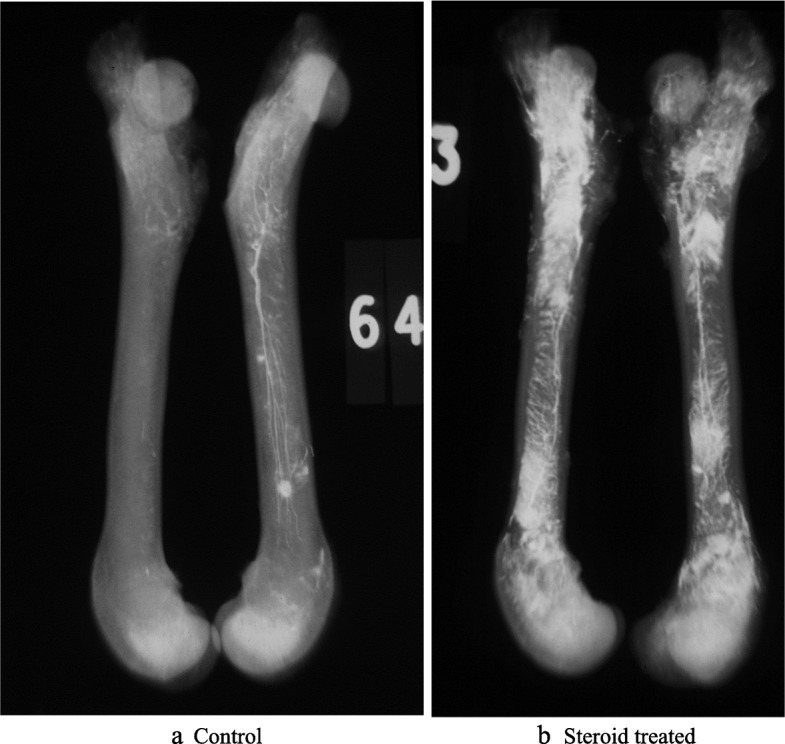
a and b showing examples of femoral angiogram microradiographs. The left radiograph 5a shows a pair of femora from a control barium angiogram. The right femoral pair 5b were from a steroid treated subject. After steroid treatment there was better barium filling
